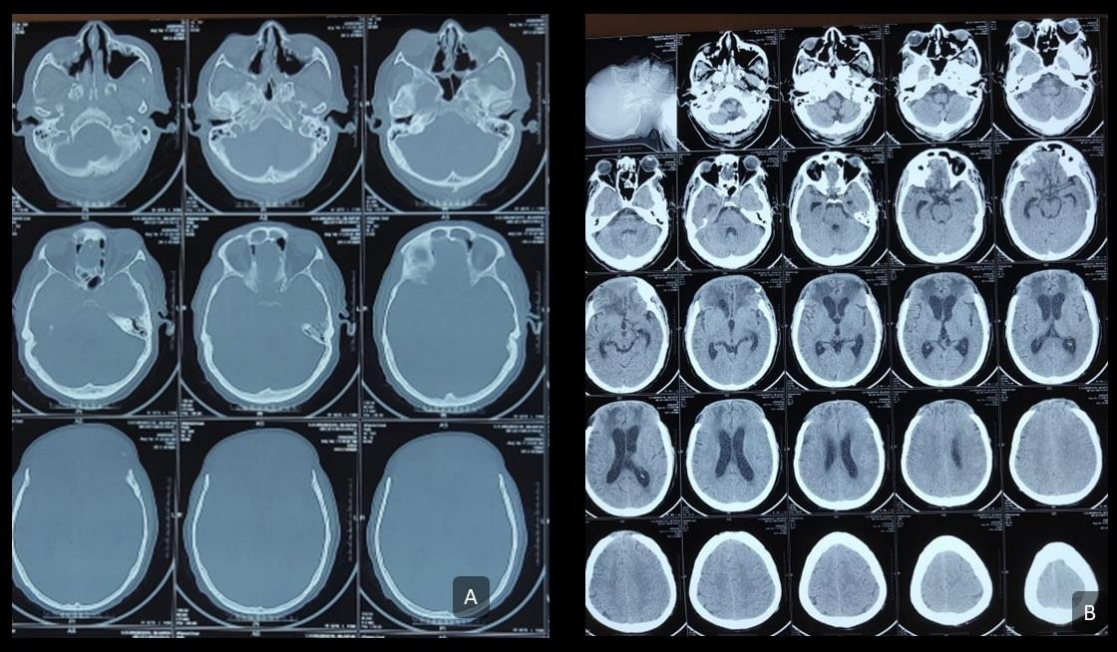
Computed Tomography Scan at 9 Months After Surgery. A: Debrided frontal bone in axial bony window cuts. B: No abnormal soft tissue density is found in the operative site, suggesting locoregional disease control.
